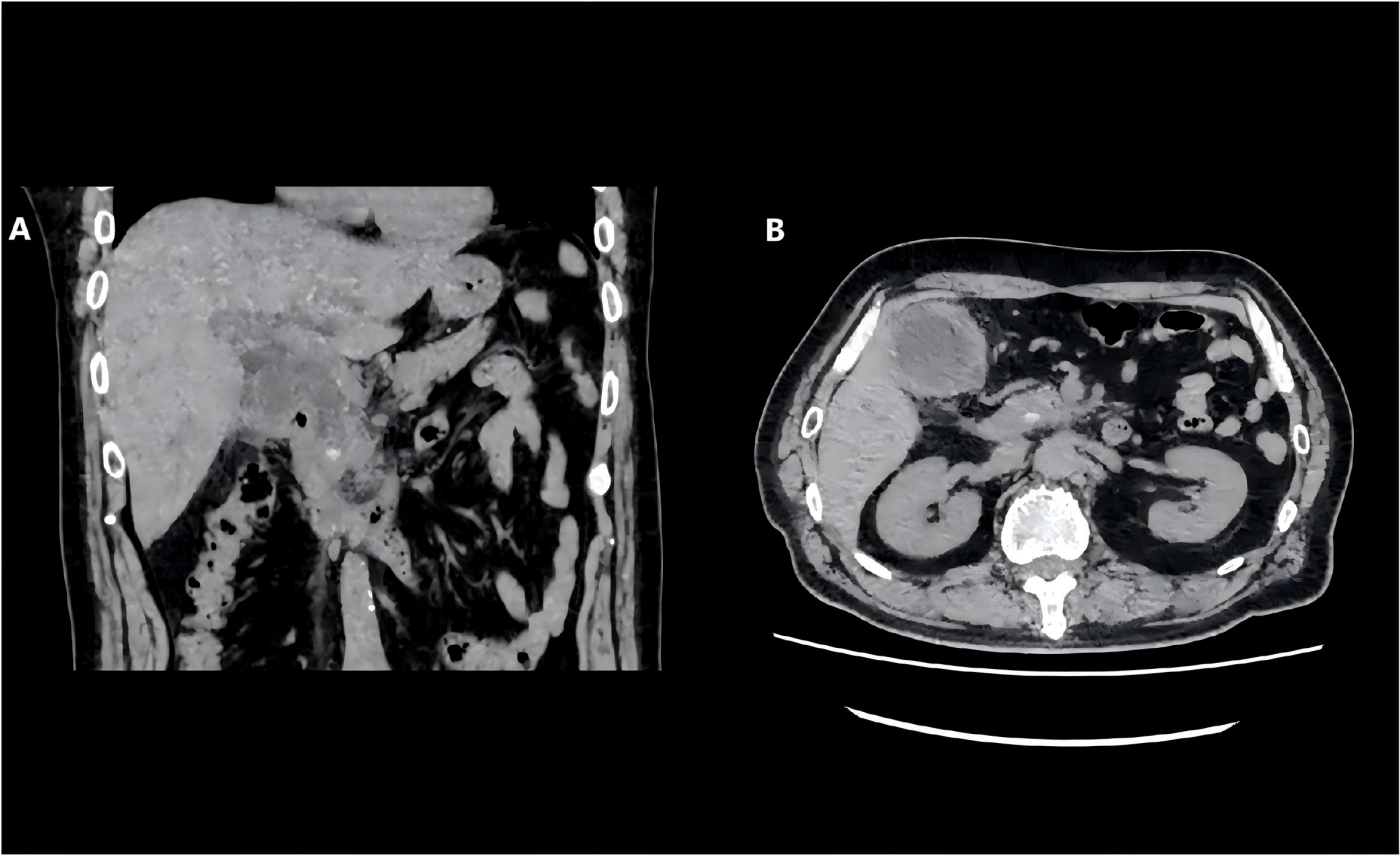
Preoperative CT scan indicated common bile duct stones, gallstones with cholecystitis. (A) Preoperative CT coronal image. (B) Preoperative CT axial image.
